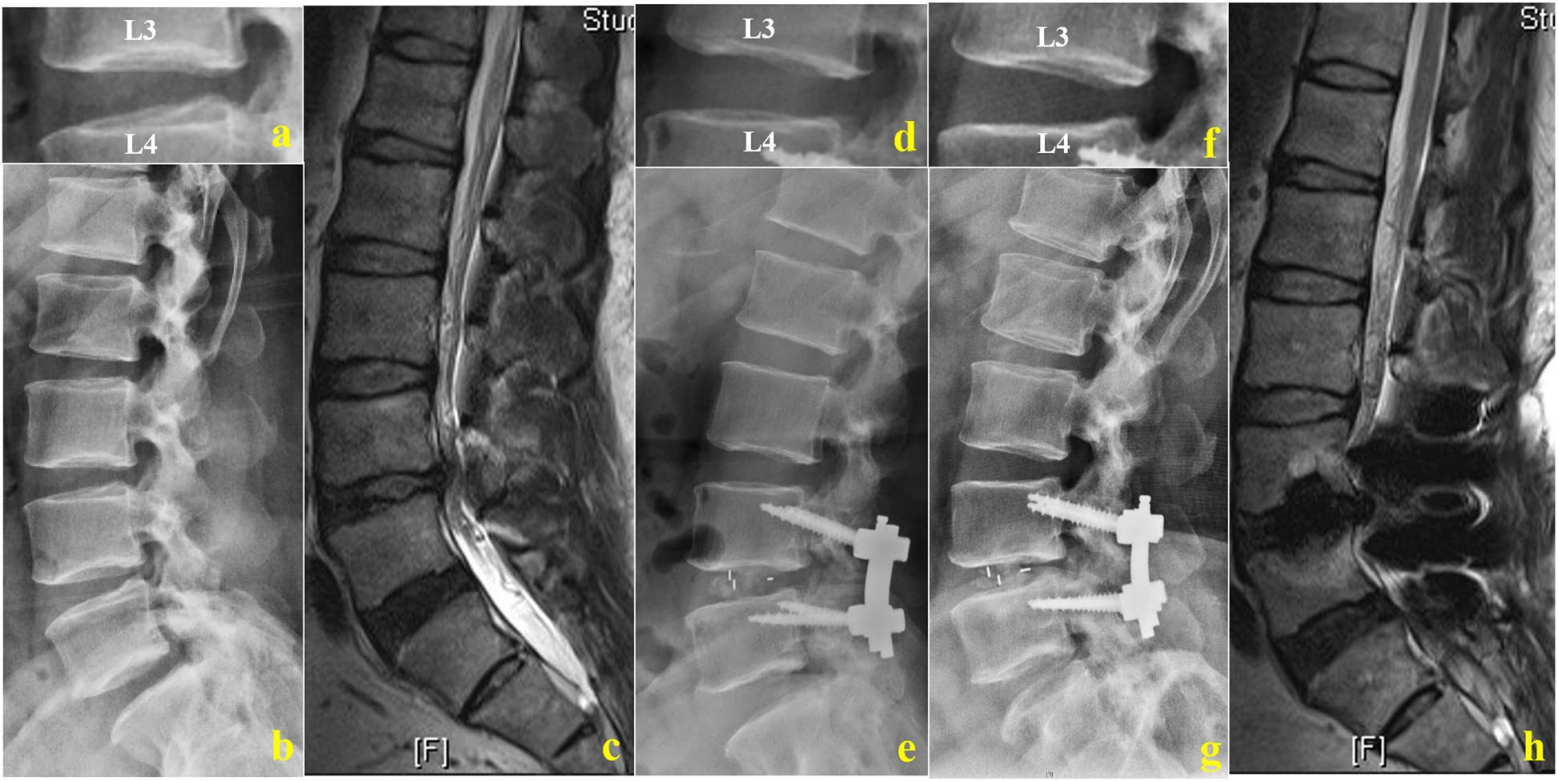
Radiological studies of a 75-year-old man with lumbar spinal stenosis after CBT-MIDLIF. a–c Preoperative plain radiographic image and preoperative sagittal magnetic resonance imaging (MRI) scan. Intervertebral space height (ISH): 14.67 mm, foraminal height (FH): 25.17 mm, foraminal width (FW): 9.98 mm, range of motion (ROM): 11.79°, lumbar lordosis (LL): 43.10°, sacral slope (SS): 36.60°, COBB 1: 8.30°, COBB 2: 18.32°, H 2/H 1: 3.18, and Pfirrmann classification of cephalic lumbar intervertebral disk: grade I. d, e Postoperative plain radiographic images at 6-month follow-up. f–h Postoperative plain radiographic image and preoperative sagittal MRI scan at 38-month follow-up. ISH: 13.60 mm, FH: 24.78 mm, FW: 10.79 mm, ROM: 12.92°, LL: 55.4°, SS: 44.70°, COBB 1: 9.10°, COBB 2: 19.15°, H 2/H 1: 3.06, and Pfirrmann classification: grade I
